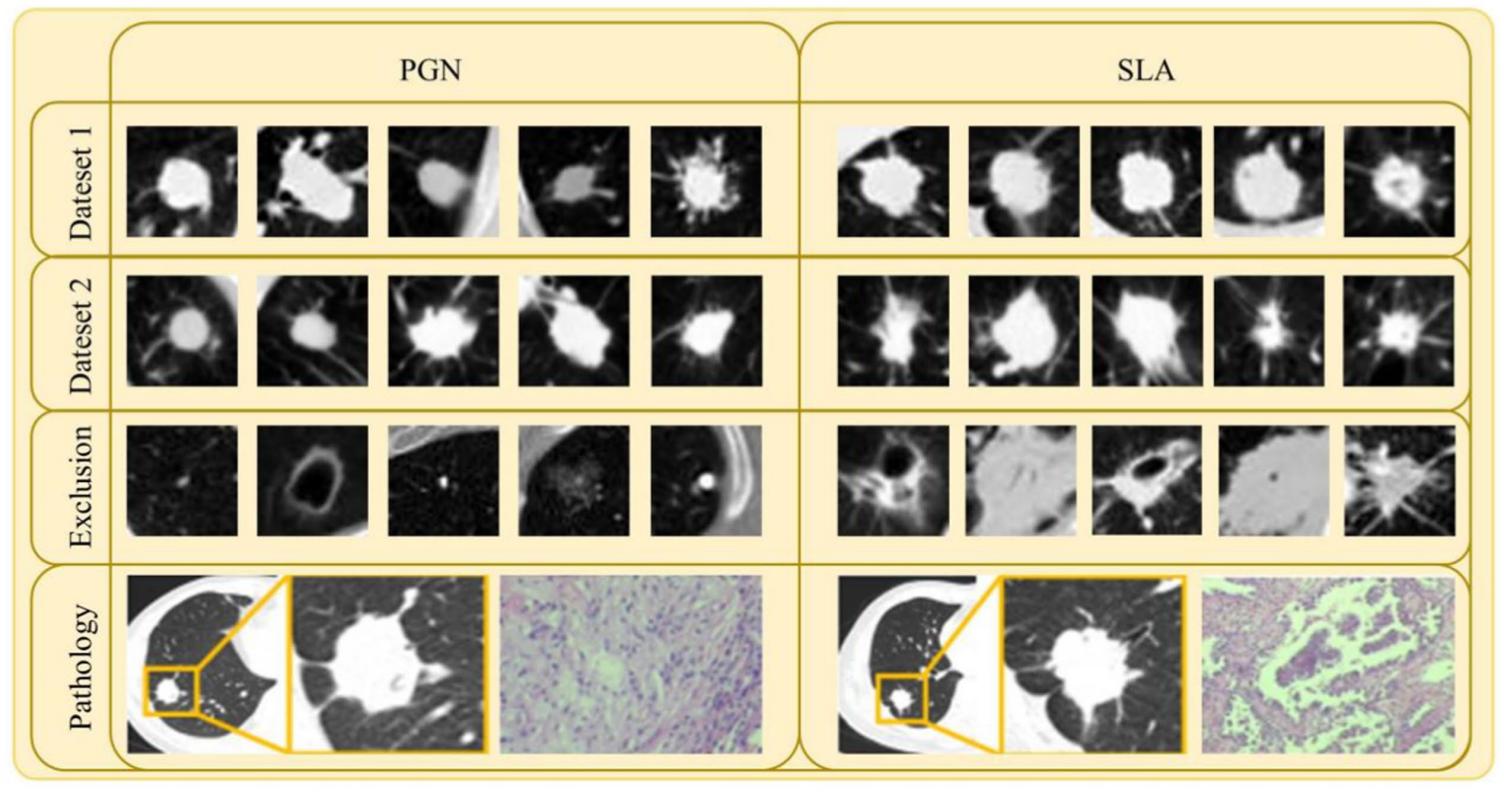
Samples of lesion images (CT and pathology) and excluded lesion images for PGN and SLA in Dataset 1 and Dataset 2.
